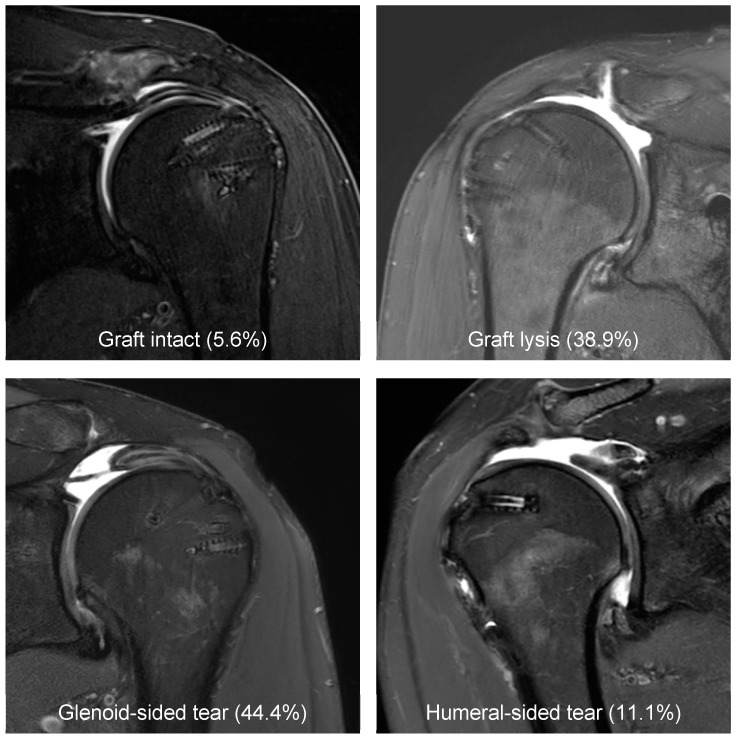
Graft integrity was evaluated with magnetic resonance imaging.
